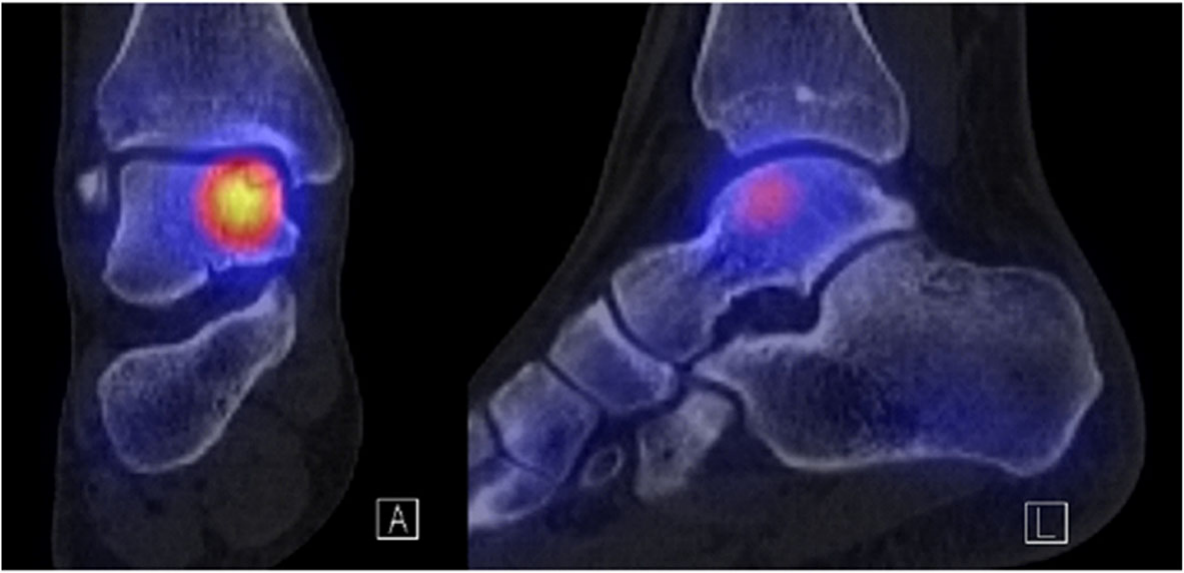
SPECT/CT of an ankle with an OCL on the talus dome. The *left* picture represents the coronal view, the *right* picture the sagittal view. Yellow indicates the highest activity in the bone, whereas in the tibia and the other foot bones the individual and physiological bone activity is shown as a *light blue*
